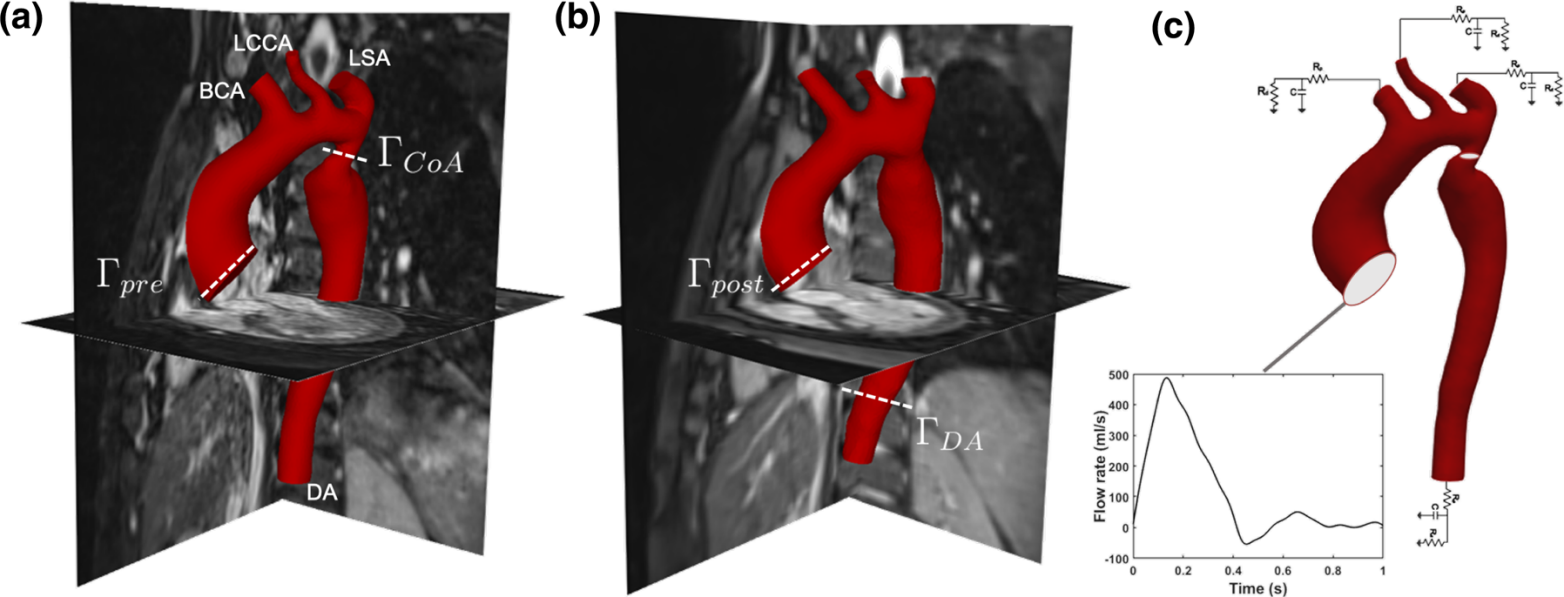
Segmented 3D geometry before ($${M_{\text{pre}}}$$) (a), and after ($${M_{\text{post}}}$$) (b) the stenting procedure with the identification of the planes used for the PC-MRI acquisitions. Time-dependent parabolic flow rate waveform (c) prescribed as inlet boundary condition at plane $$\Gamma _{\text{pre}}$$ before, and at plane $$\Gamma _{\text{post}}$$ after the stenting procedure. The 3WKMs are coupled at the four outlets: Brachiocephalic Artery (BCA), Left Common Carotid Artery (LCCA), Left Subclavian Artery (LSA) and Descending Aorta (DA).
